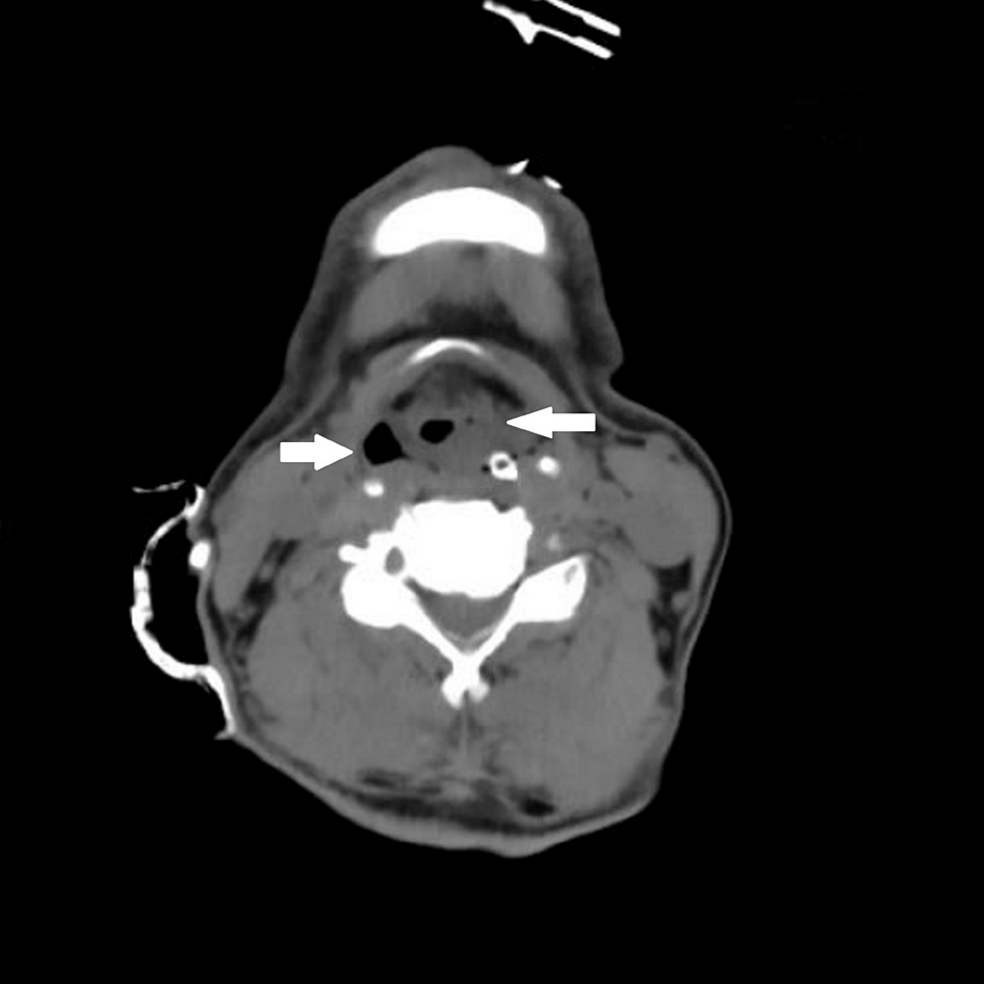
CT of the neck revealing soft tissue edema with significant airway compromise
